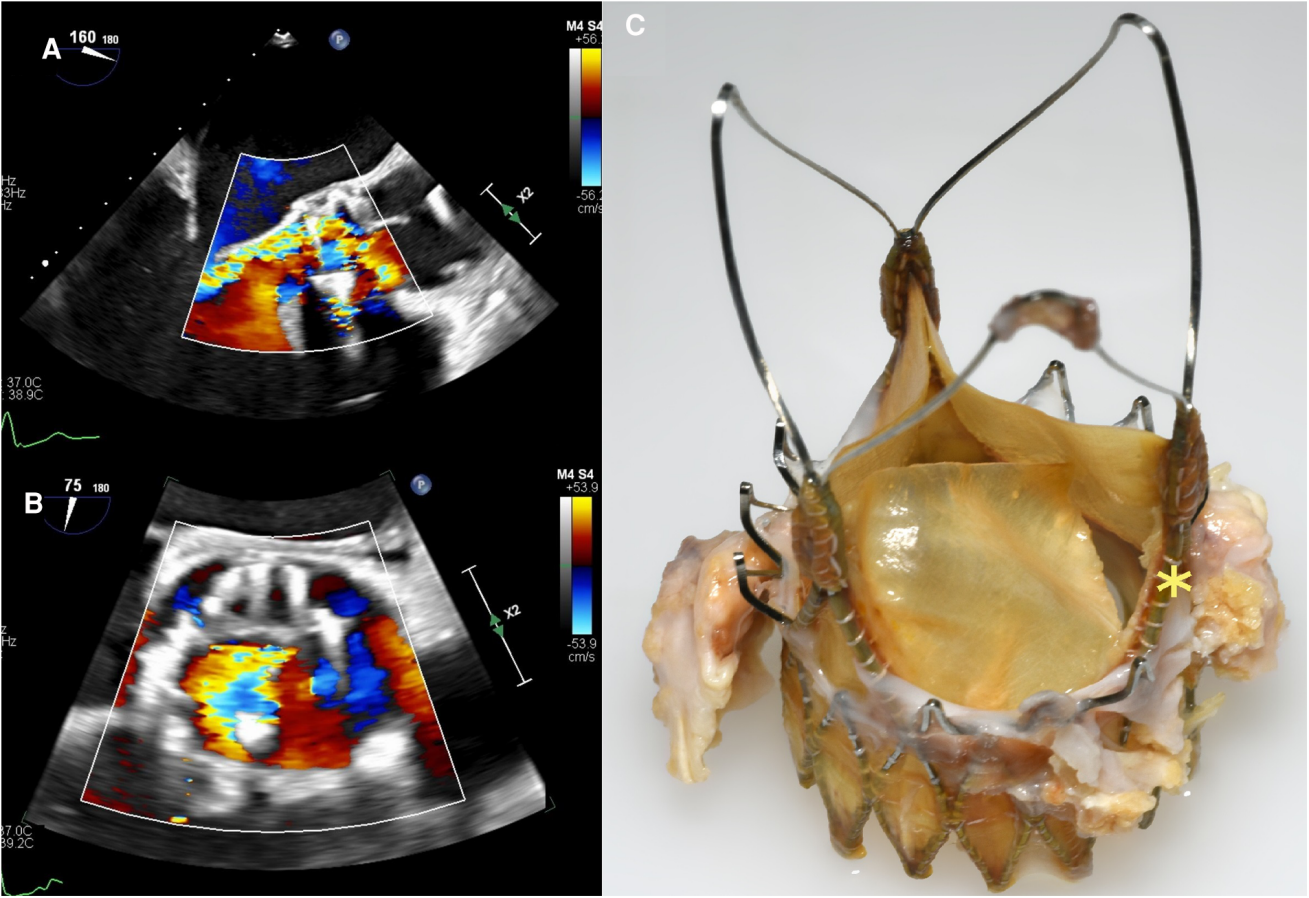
Case 1: transesophageal echocardiography three-chamber view with color-flow Doppler (A) and aortic valve-centered short-axis view with color-flow Doppler (B) showing an eccentric, severe intraprosthetic regurgitation. (C) Explanted ACURATE Neo prosthesis with leaflet disruption with its adjacent strut in the noncoronary position (yellow asterisk).
